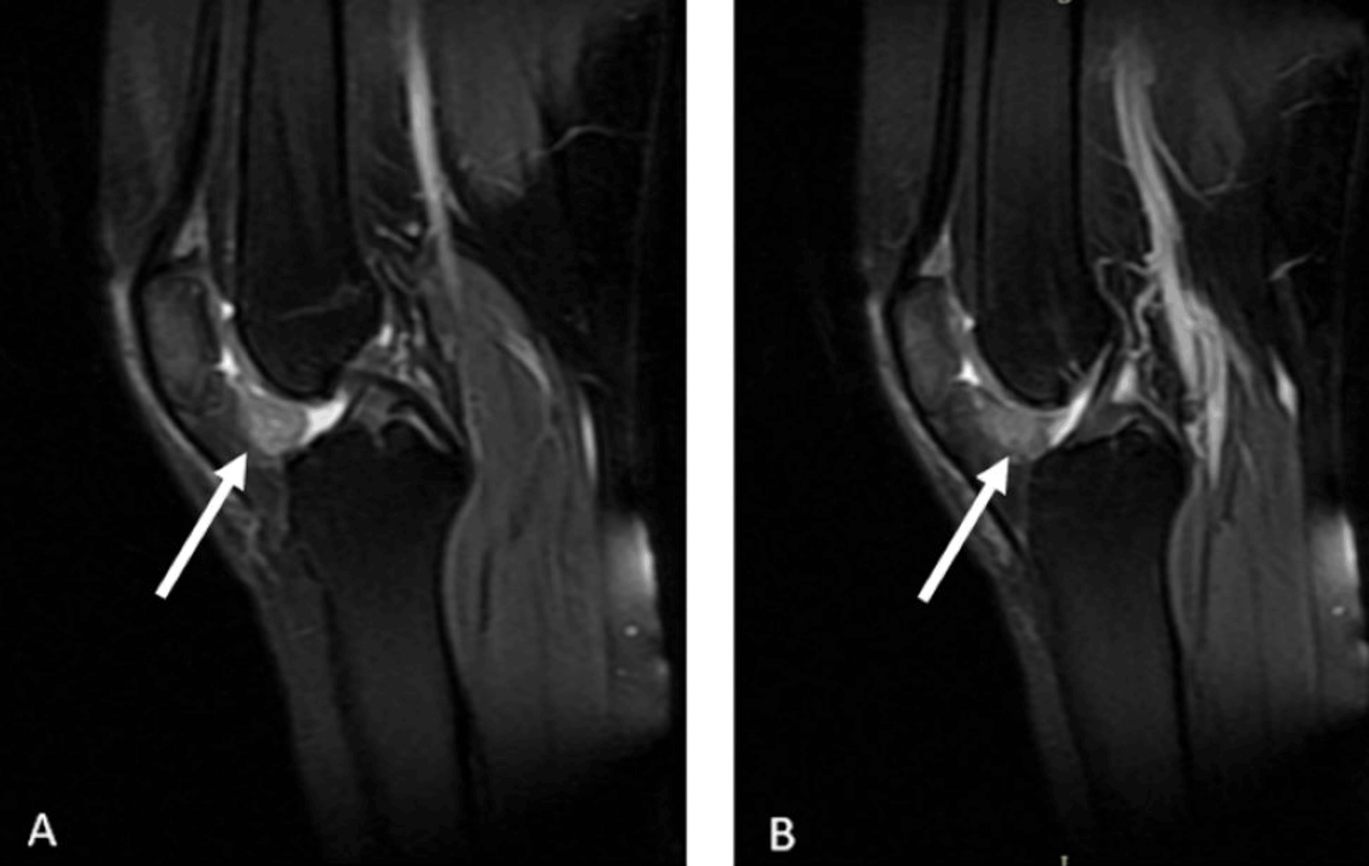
The patient's T2-weighted sagittal MRI sections. (A) The lesion is clearly visible within the affected region, demonstrating hyperintense characteristics. (B) Another sagittal section, showing the extent and morphology of the lesion The white arrows indicate the lesion MRI: magnetic resonance imaging
